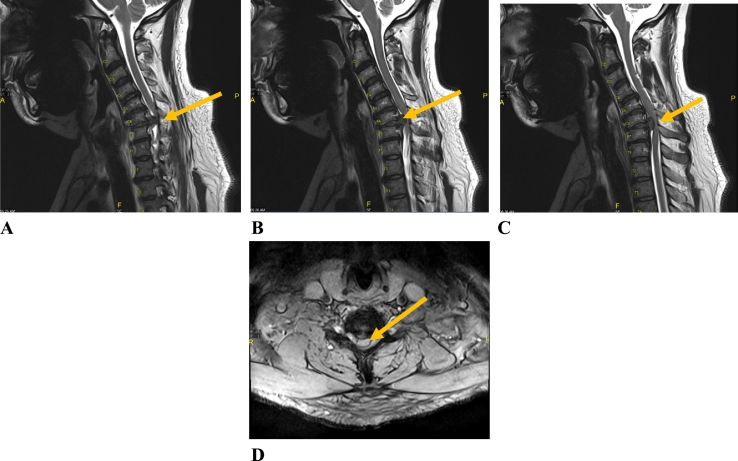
MRI of the cervical spine (lateral view, A, B and C) and C6–C7 (axial view, D) showing significant disc herniation at two levels (C5–C6 and C6–C7) with no spinal cord myelomalacia.
